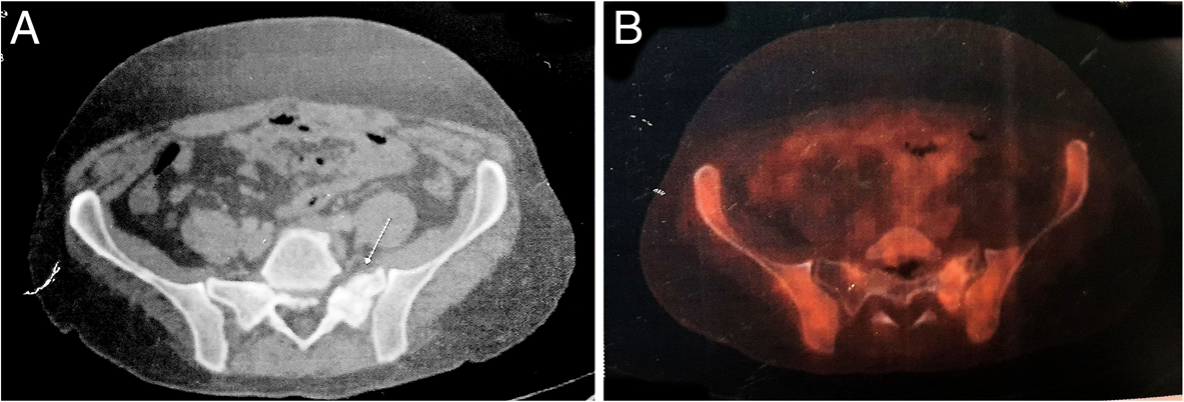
FDG-PET/CT in 2017 after 6 cycles of R-CHOP regimen. a Sclerotic lesion in left ala of the sacrum. b Mild FDG uptake in the left ala of sacrum (SUV of 3.22)
